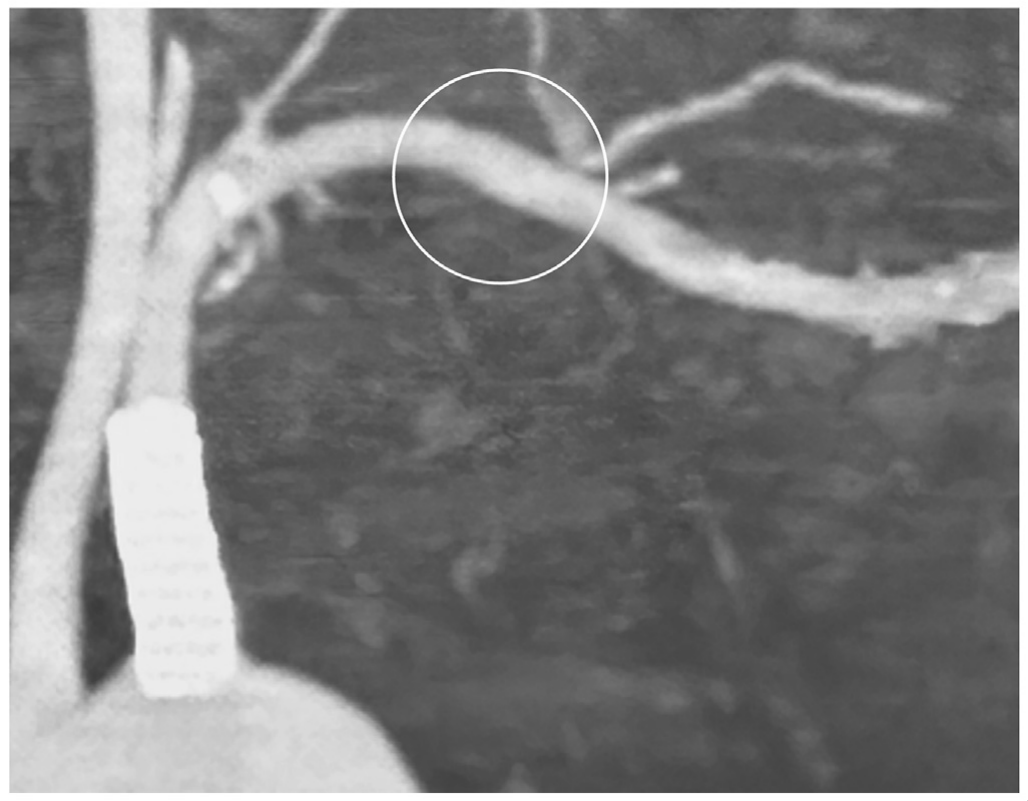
Postoperative computed tomography (CT) angiography with the arm elevated, showing an improvement of the subclavian artery stenosis at the costoclavicular space.
